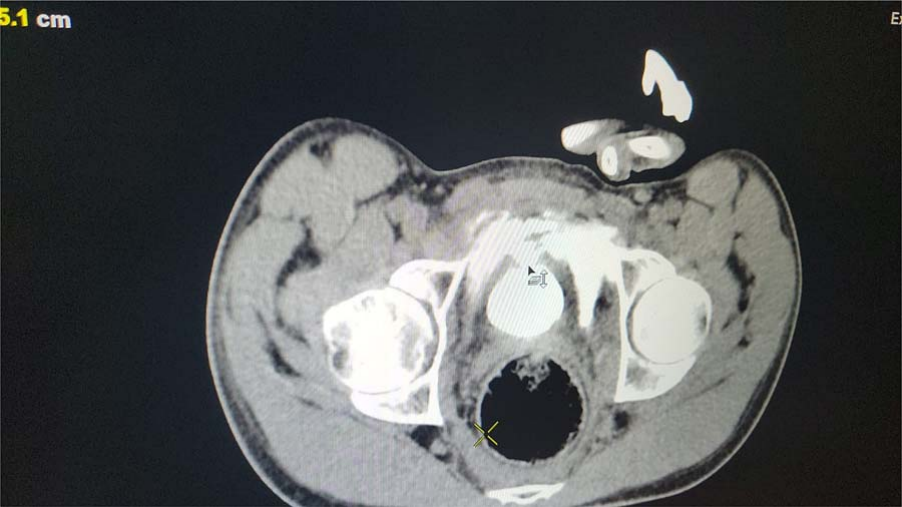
CT scan reveals contrast material leaking from the bladder lumen into the surrounding pelvic cavity, indicating a bladder rupture.
